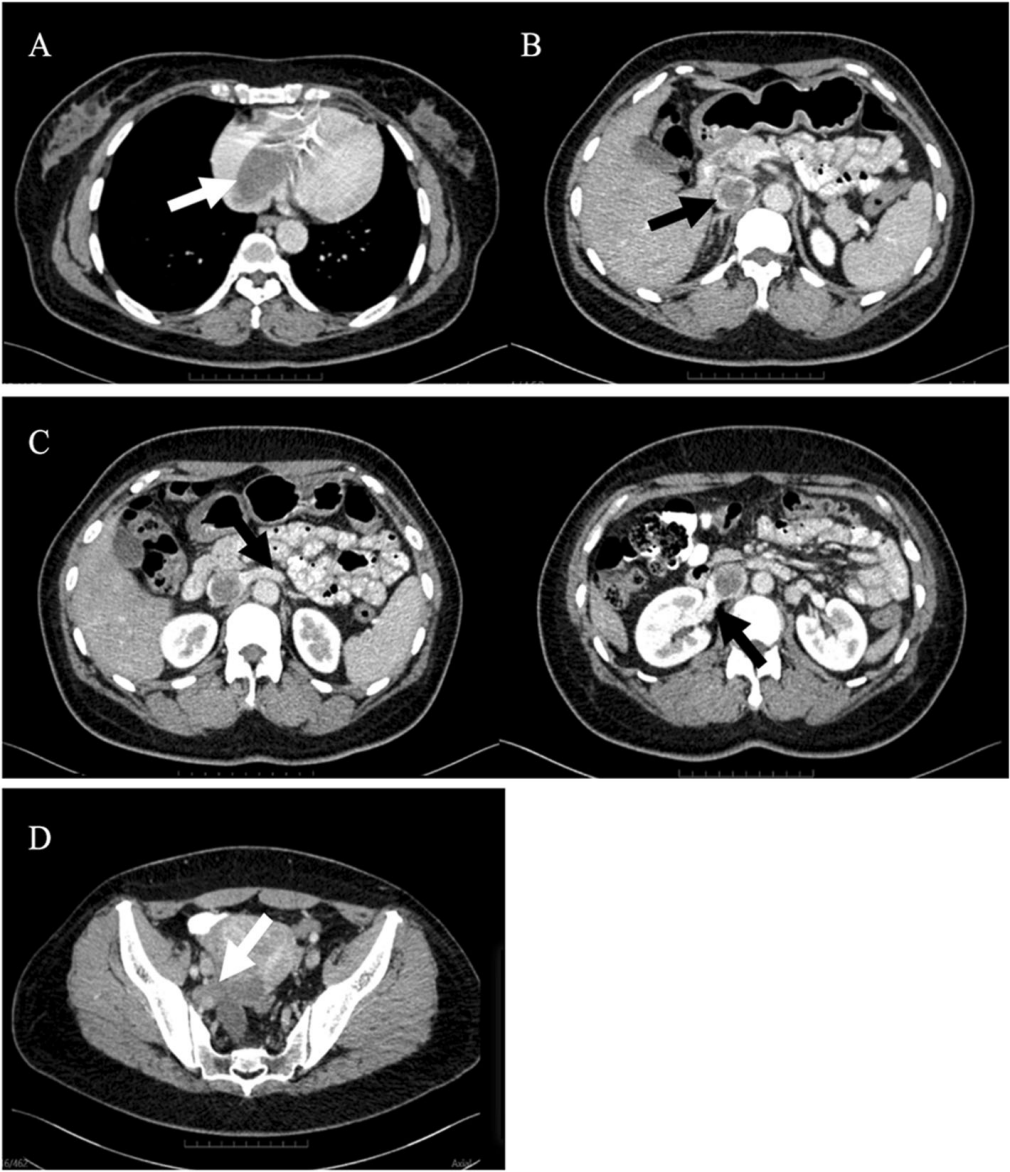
Axial slice of the CT image shows the shape and route of extension of the intravenous leiomyomatosis. a This image shows that the tumour grew into the right atrium and extended to the right ventricle; b This image shows that the tumour grew along the inferior vena cava, resulting in enlargement of the vein; c These two images show that the bilateral renal veins were not invaded; d This image shows that the leiomyoma grew into the vein through the right uterine vein and internal iliac vein
